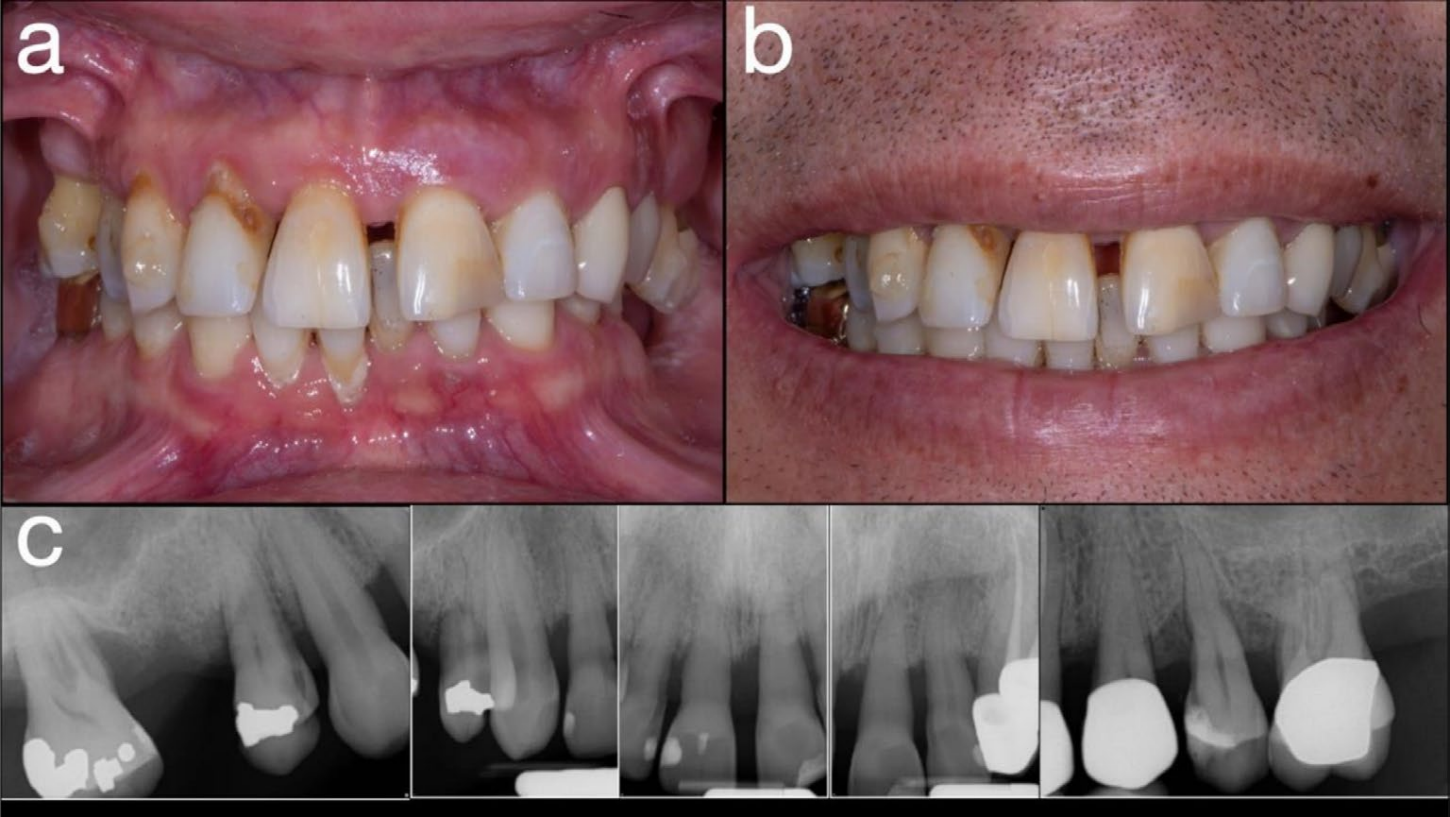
(a) Frontal view in situ; (b) smile view in situ; and (c) periapical radiograph of the maxillary dentition.
